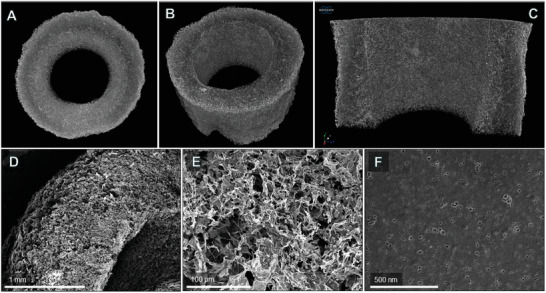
Micro‐CT 3D imaging (A‐C) and FESEM analysis (D‐F) of tubular freestanding carbons made of ChiSuc recipe, frozen in the designed brass mold (Figure S5C, Supporting Information) and carbonized at 1000 °C.
